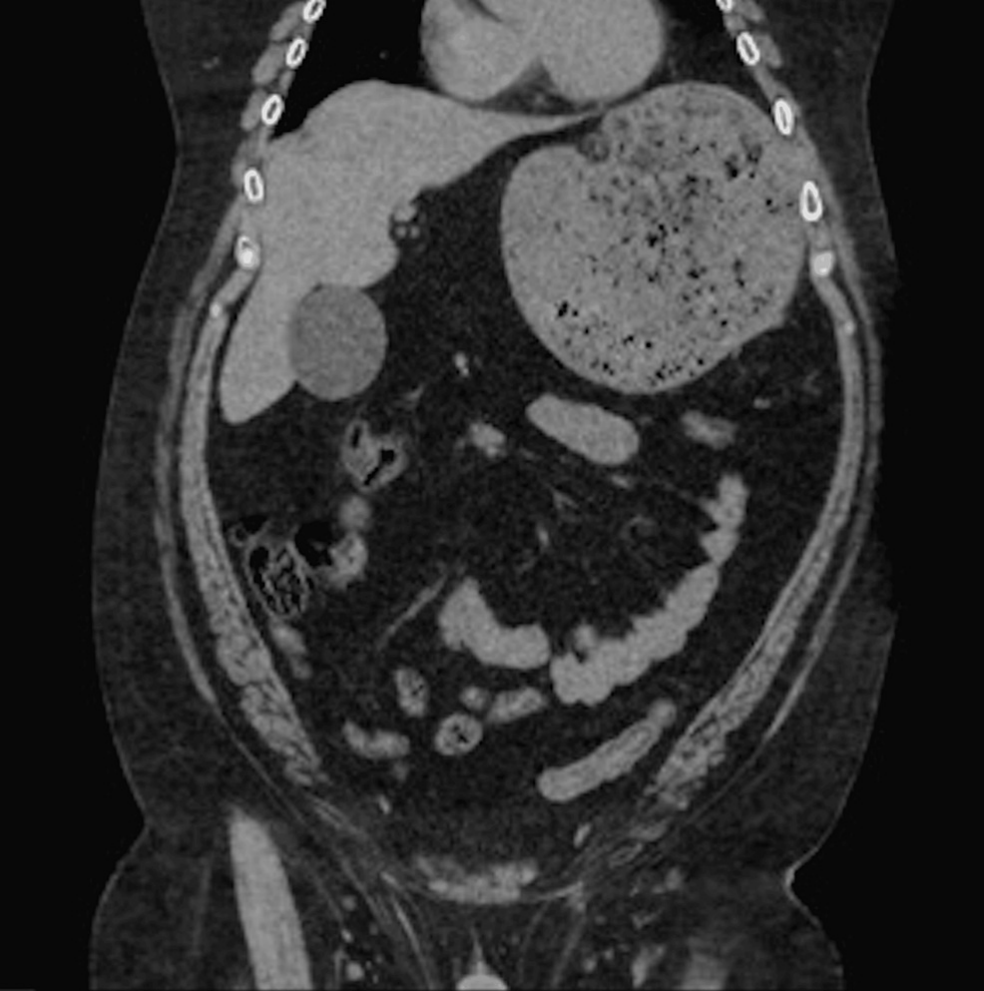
Coronal CT before decompression of gastric volvulus
